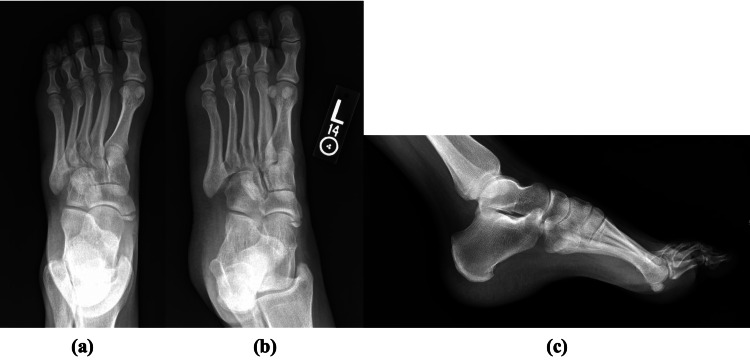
Non-weight bearing anteroposterior (a), oblique (b), and lateral radiographs (c) of the left foot pre-operatively.
